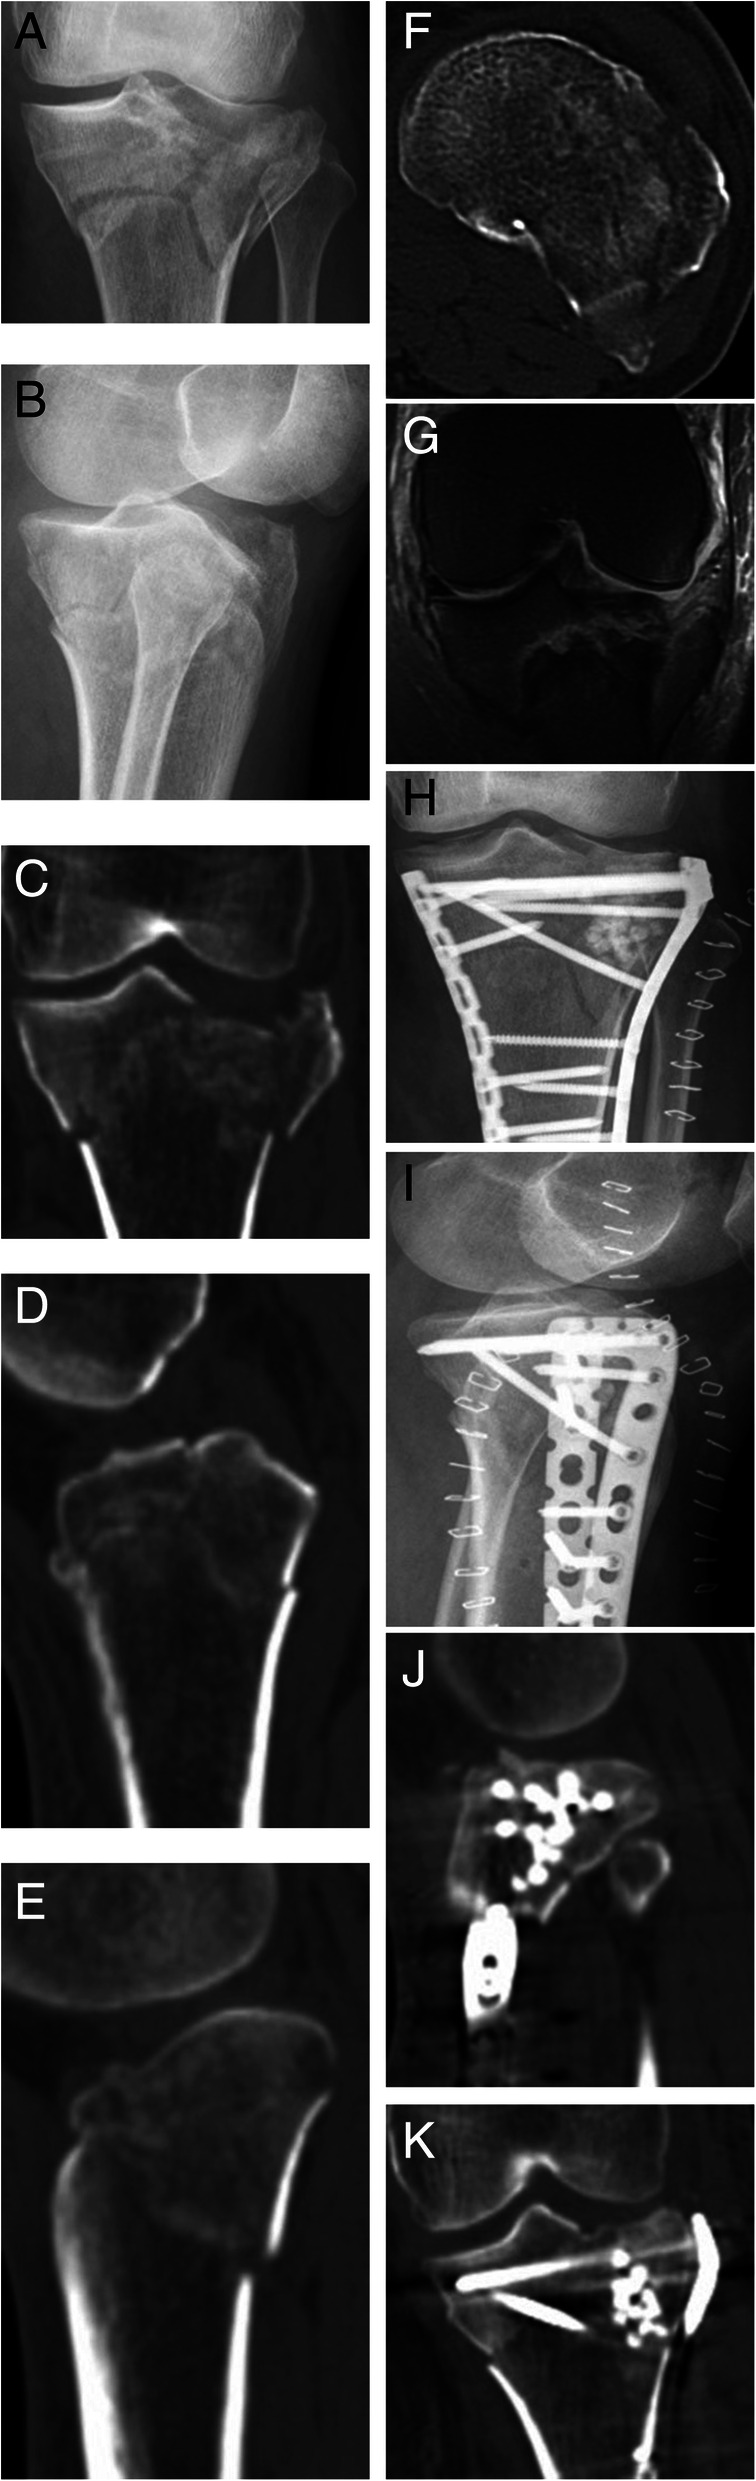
F, 57Y, medial, and lateral plateau fractures with MCL injury. a, b Preoperative x-ray shows the medial and lateral tibial plateau fractures and significant change in pTSA. c–f Preoperative CT scans show collapse and splitting of the bilateral plateau fractures and the anteverted plateau. g Preoperative MRI shows medial collateral ligament injury. h–k Postoperative x-rays and CT show anatomical fracture reduction and the artificial bone graft
